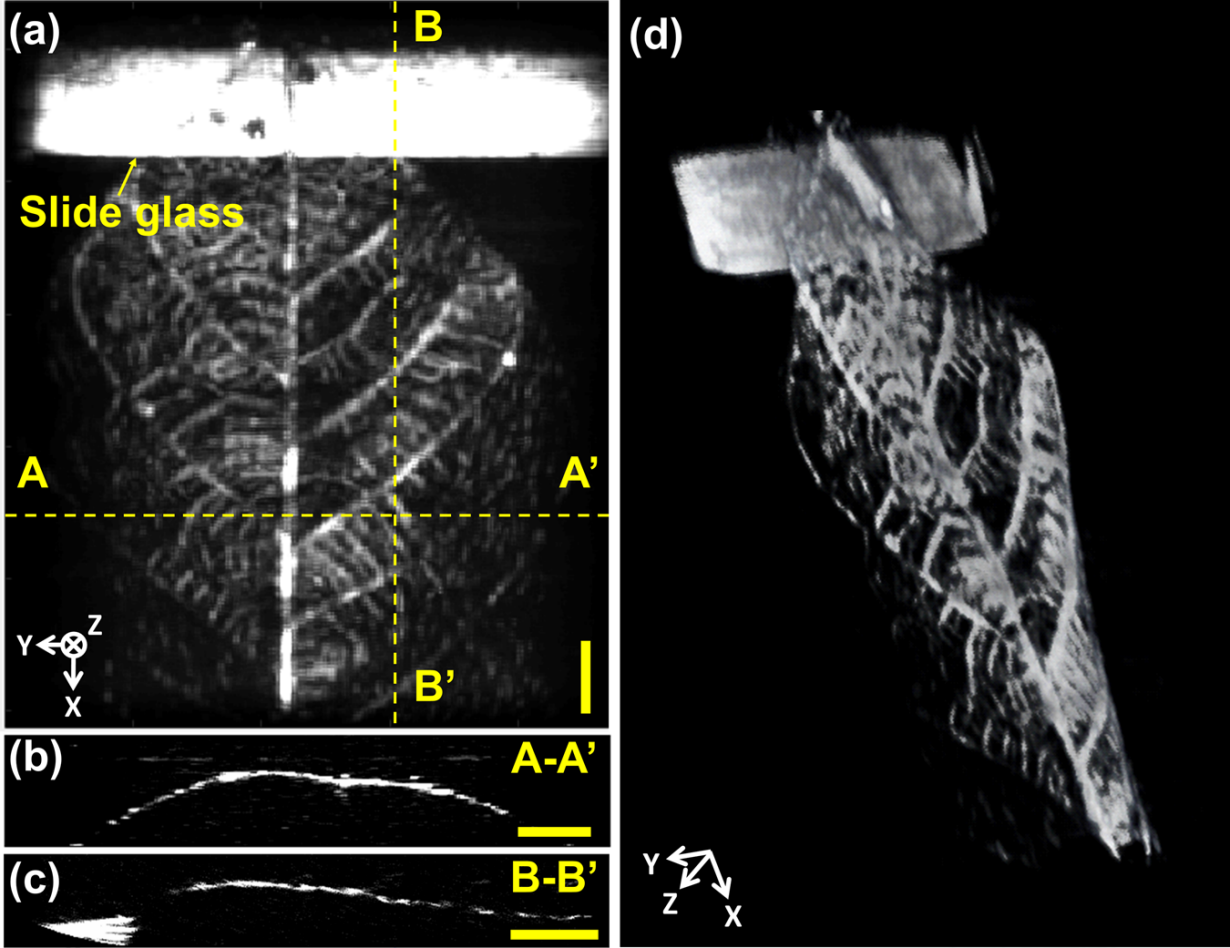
3D volumetric ultrasound imaging of a leaf skeleton. (a) Maximum intensity projection (MIP) image. (b) Global cross-sectional B-mode images along (b) the line A-A’ and (c) the line B-B’. (d) 3D rendered image. Supplementary Movie S1. All scale bars are 3 mm.
